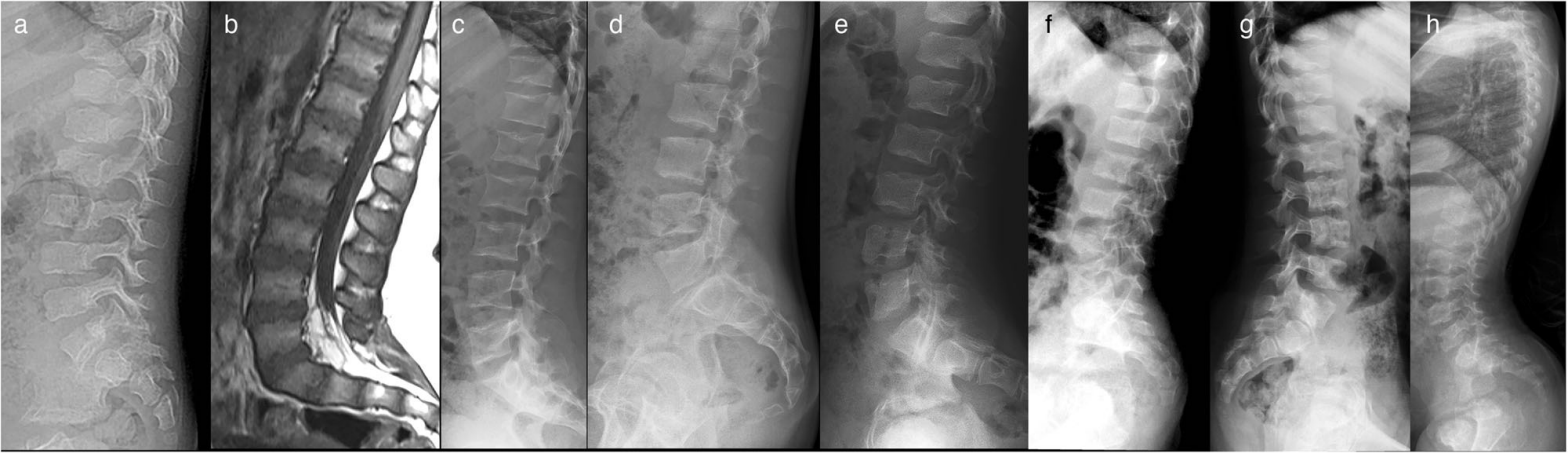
Lateral radiographs and sagittal MRI of the lumbar spine. a Radiograph of Patient 1-III:4 at age 3 years showing mild platyspondyly. b MRI of Patient 3 at age 7 years showing mild platyspondyly with irregular end plates and lumbosacral lordosis. c Radiograph of Patient 4-II:1 at 18 years of age showing mild platyspondyly with endplate modification. d Radiograph of Patient 4-II:4 at 15 years of age showing mild modification of vertebral end plates. Platyspondyly is not seen. e Radiograph of Patient 5-III:1 at age 7 years showing mild platyspondyly with severe lumbosacral lordosis and irregular end plates, and short neural arches of the lower lumbar spine and spinal canal stenosis. f Radiograph of Patient 6-II:1 at 7 years old, showing subtle irregularities of the vertebral end plates. g Radiograph of Patient 7-III:2 at 8 years showing vertebral bodies with central notches and anterior ossification defects. h Radiograph of Patient 7-III:3 at 4 years showing increased lumbosacral lordosis and vertebral bodies with anterior ossification defects and irregular end plates.
